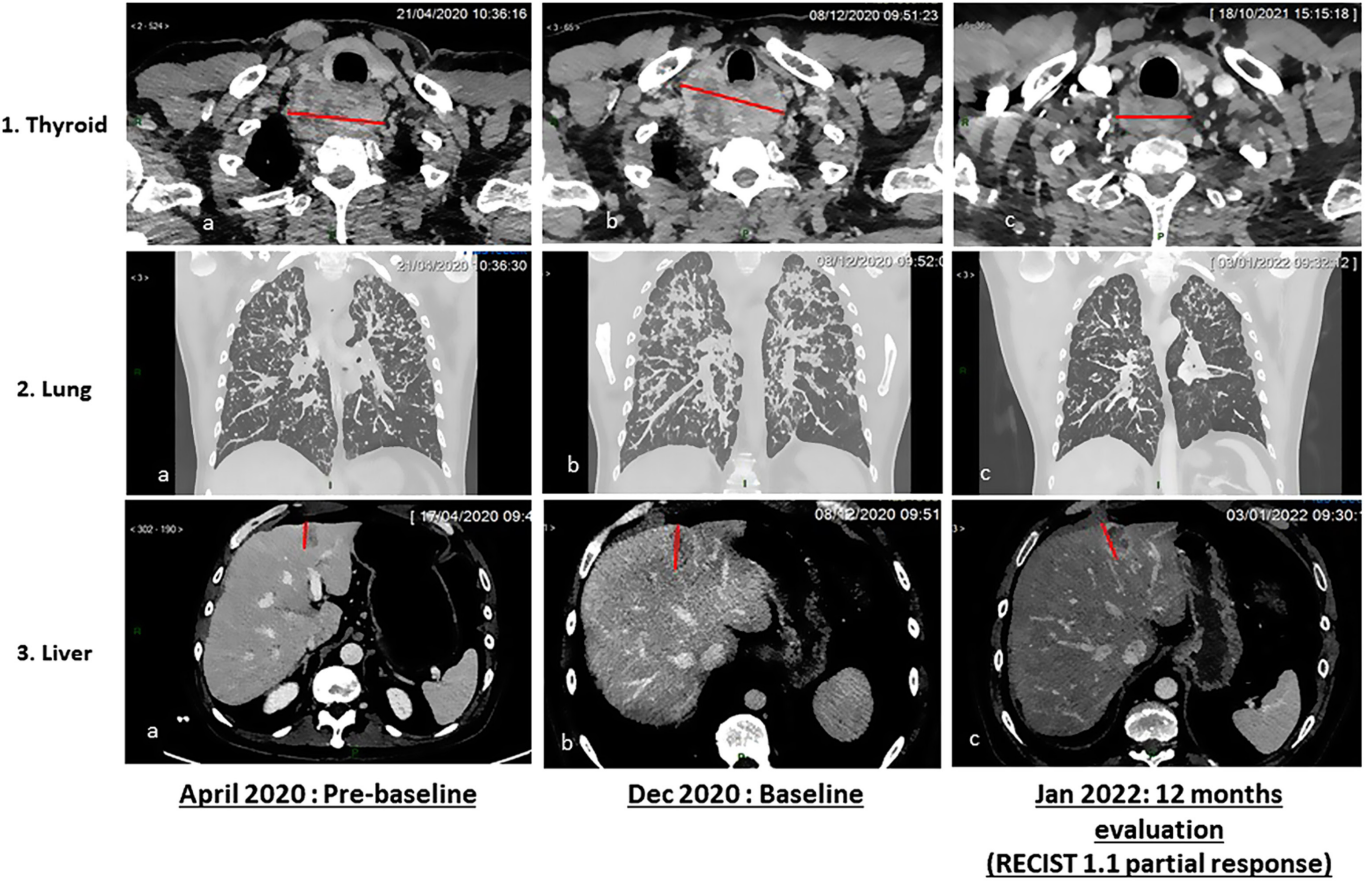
RECIST 1.1 partial response and biochemical response with Pralsetinib. 1. (A, B, and C) Axial CT slices showing a thyroid mass with retrotracheal extension. At 12 months, decrease in size and enhancement of the mass. 2. (A, B, and C) CT scan with coronal reconstruction in maximum intensity projection (MIP) showing diffuse bilateral tiny nodules. The nodules increase in number and size between pre-baseline and baseline examination and decrease significantly at 12 months. 3. (A, B, and C) Axial CT slices showing a liver met in segment III. At 12 months, there is a small reduction in the size of the lesion. However, we note the appearance of a central hypodense zone related to a marked reduction in its vascularization.
